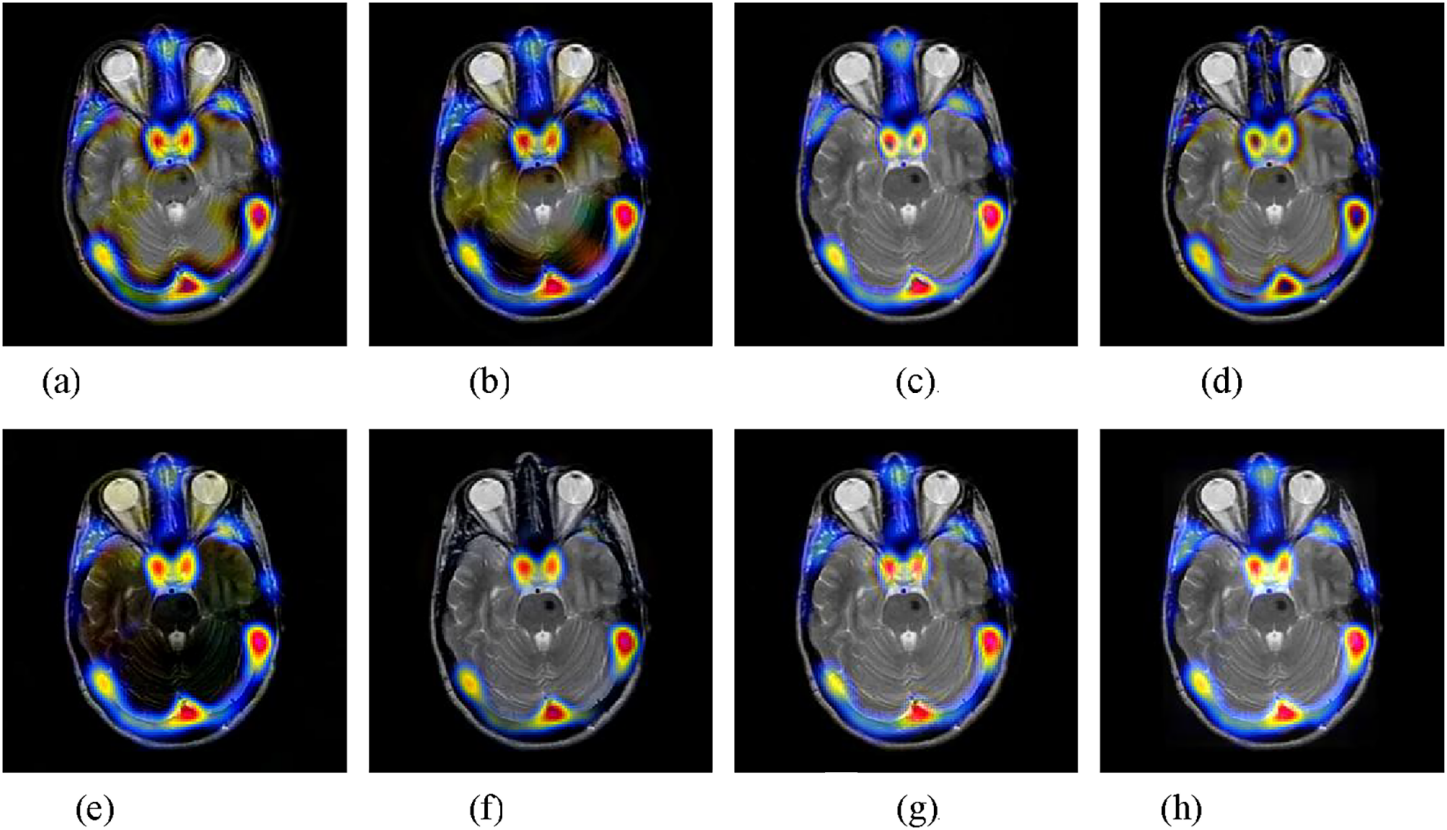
Fusion results of different methods for MRI and SPET images. (a) CNN-NSCT. (b) NSST. (c) NSST-ST. (d) SR. (e) MST-SR. (f) GFF. (g) DSWT-RBFN. (h) Our method.
